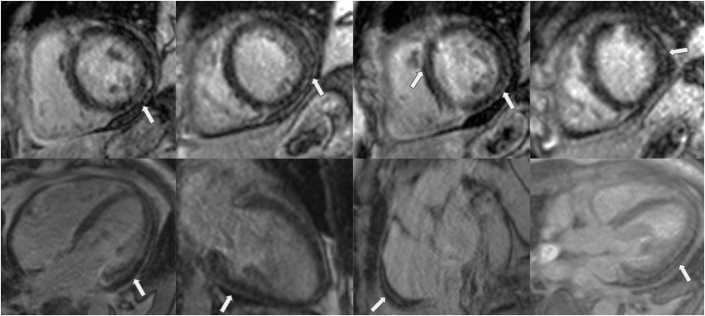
Cardiac Magnetic Resonance Imaging on Day 11 Cardiac magnetic resonance imaging obtained on day 11 demonstrating mildly dilated left ventricle and patchy subepicardial and mid-myocardial late gadolinium enhancement in 4 segments, consistent with acute myopericarditis. T2-weighted imaging showed increased myocardial signal intensity. White arrows indicate area of myocardial late gadolinium enchantment.
